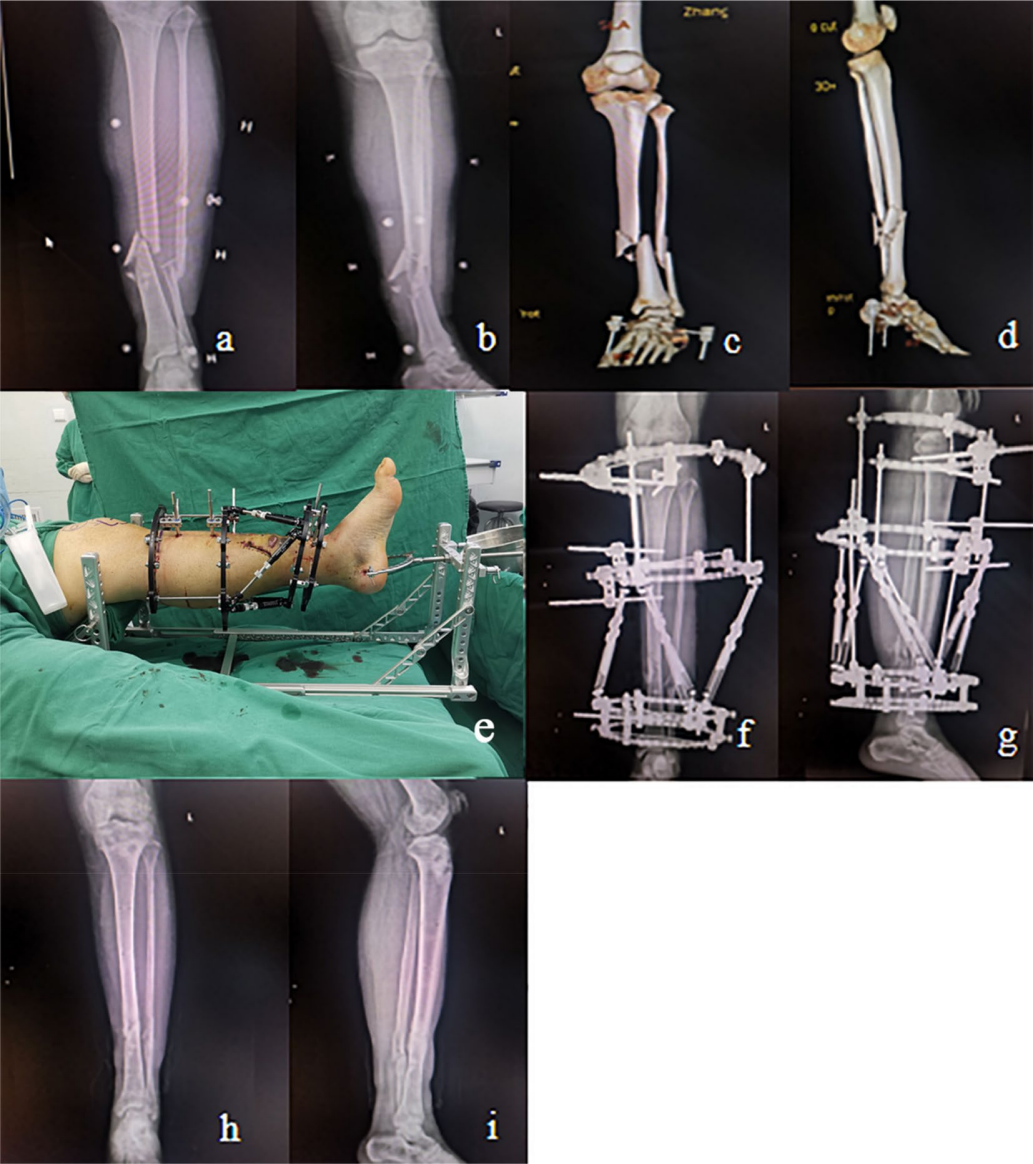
A 45‐year‐old male with Gustilo–Anderson classification IIIB open tibial shaft fracture (OTA/AO 42‐B3, highly comminuted) treated with double reverse traction‐assisted closed reduction and HEF. (a, b) Preoperative AP and lateral radiographs showed significant displacement, shortening, and comminution. (c, d) Preoperative 3D‐CT reconstructions further delineate the spiral‐oblique fracture pattern with butterfly fragments. (e) Intraoperative setup identical to Figure 3. (f, g) Postoperative AP and lateral radiographs at 1 week showing satisfactory reduction with restoration of length, alignment, and minimal residual displacement. (h, i) Final follow‐up radiographs 1 week after HEF removal (6.5 months postoperatively) demonstrating complete fracture union with good cortical continuity and alignment.
